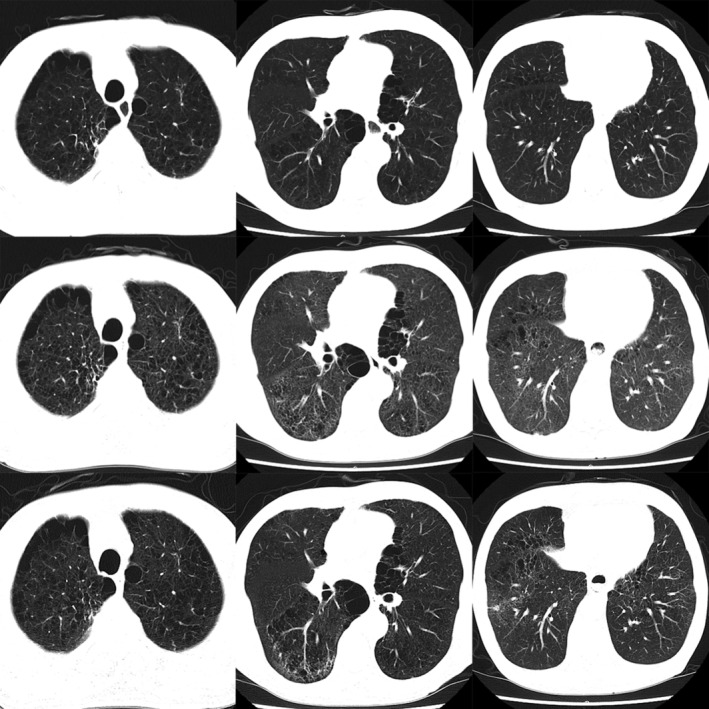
Chest computed tomography (CT) findings. Upper row is before the onset of symptoms, middle row is during presentation, and lower row is after 14 days of prednisolone therapy. Chest CT at onset revealed ground‐glass opacities in bilateral lung fields, which disappeared after prednisolone therapy.
